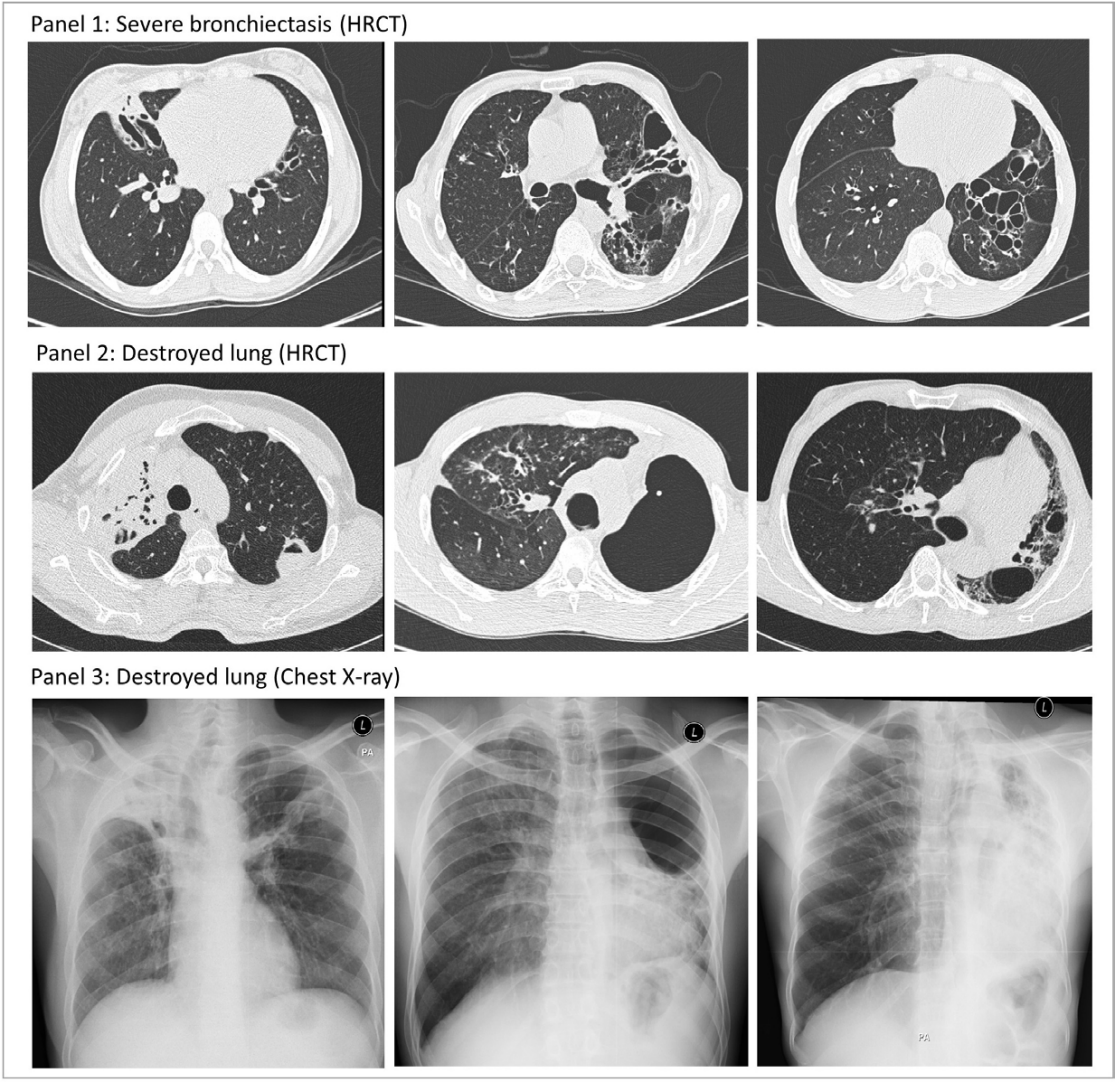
High resolution CT (HRCT) and chest x-ray imaging from participants with severe bronchiectasis (panel 1) or destroyed lung (panels 2 and 3, paired imaging from the same individuals), captured at TB-treatment completion. Bronchiectasis: Airway lumen diameter greater than accompanying pulmonary artery outer diameter, or airways visible <1 cm of lung periphery, or lack of normal airway tapering. Destroyed lung: ≥1 lung lobe in which ≥90% of parenchyma occupied by atelectasis or parenchymal banding, or destroyed by cavities/cystic airspaces.
